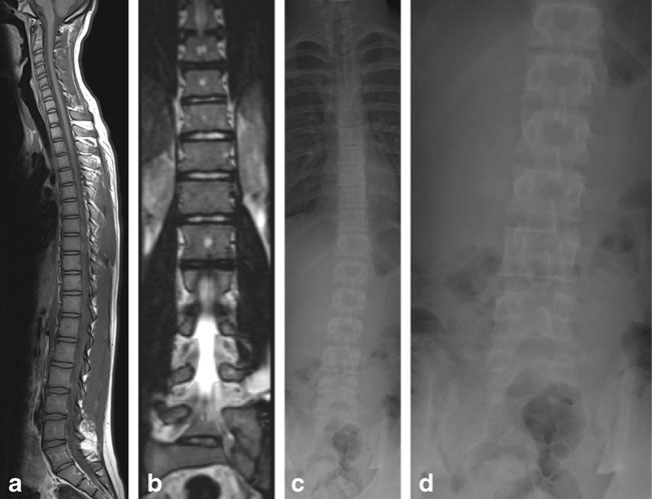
15-year-old boy with back pain. Sagittal T1-weighted image (a) and coronal reformats of a sagittal SPACE sequence (b) demonstrate a transitional lumbosacral vertebra. Counting from the top, this is a lumbarised S1 vertebra. This can also be identified on an anterior-posterior (AP) radiograph (c) of the whole spine, better appreciated on a magnified view of the lumbar spine only (d). The only reliable method for determining the nature of a transitional vertebra is to count from the top
